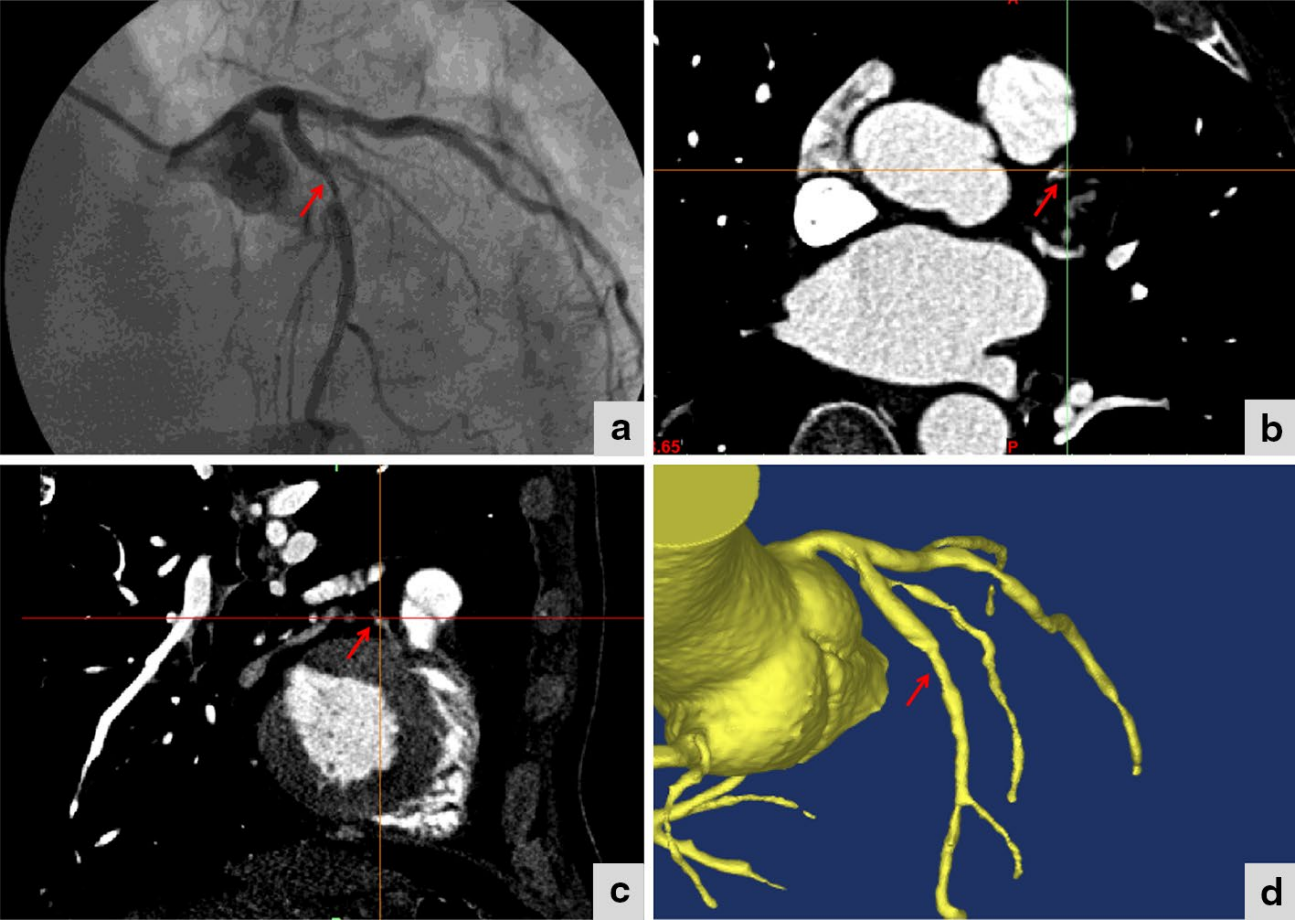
DSA, 2D CTA, and 3D CTA datasets of the same luminal stenosis. A coronary arterial segment with luminal stenosis (marked with *an arrow*) by DSA criteria is shown (a). Corresponding segment on 2D CTA in short axis (b) and in long axis (c) is shown at the position with the greatest degree of the stenosis. Corresponding segment on 3D CTA datasets (d).
